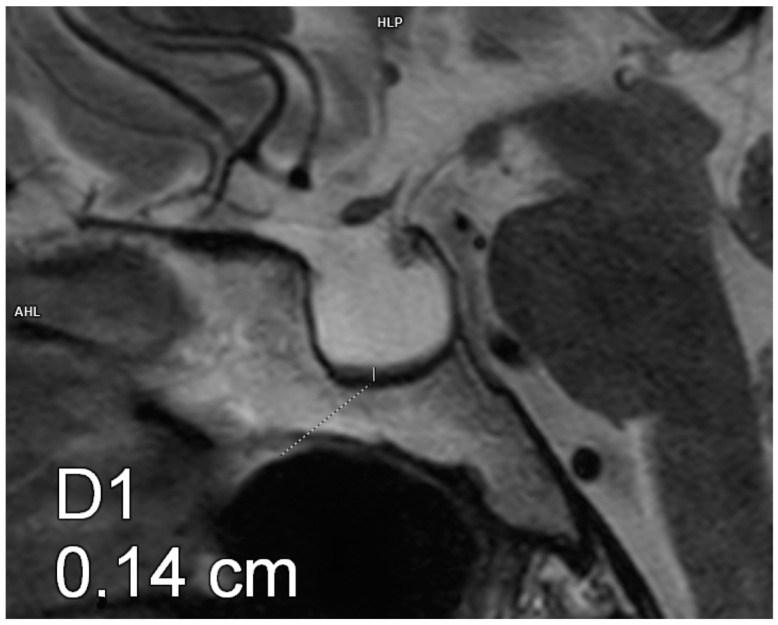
Empty sella. Magnetic resonance imaging, T2-weighted image, sagittal plane. The craniocaudal diameter of the pituitary gland is 1.4 mm.
